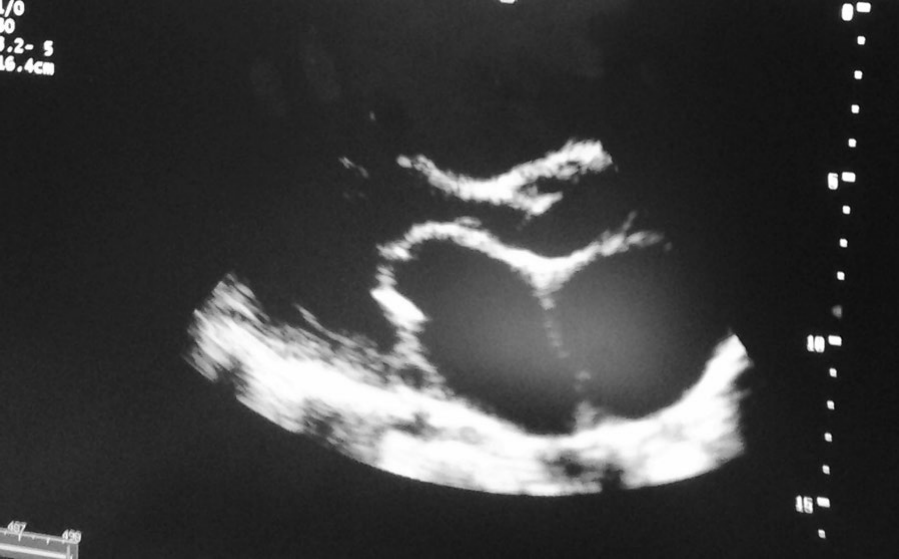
Echocardiogram of a subject with rheumatic mitral stenosis
